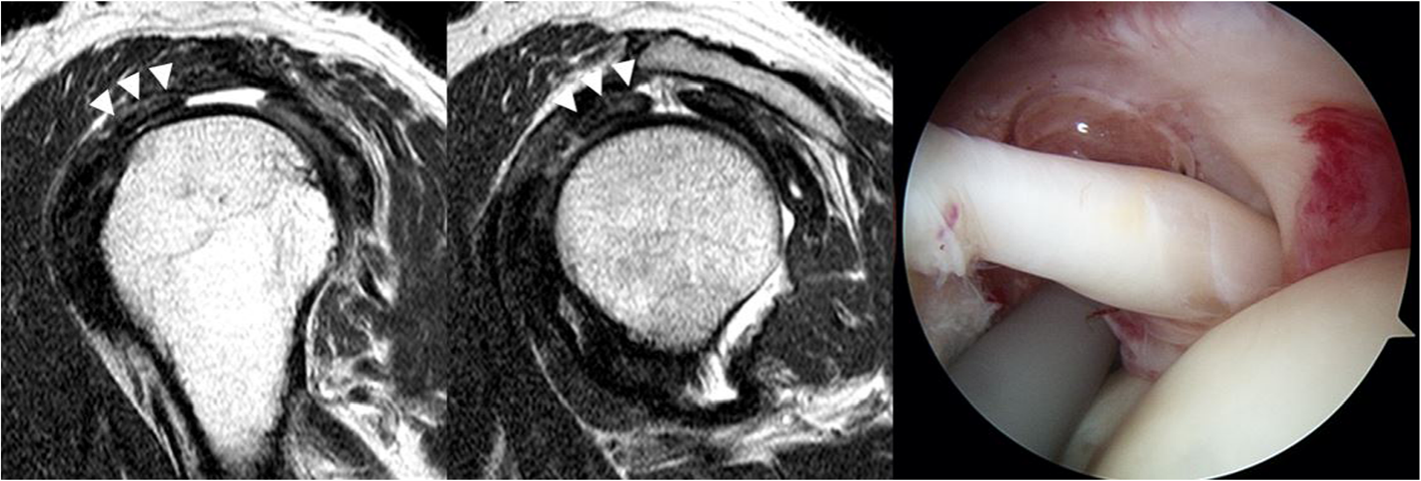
Two consecutive T2-weighted parasagittal MRI showing abrupt diameter change and high signal intensity inside of the tendon (white arrowhead). The contour of the tendon was smooth and uniform. This patient’s MRI revealed hypertrophy in the long head of the biceps tendon (Type II)
